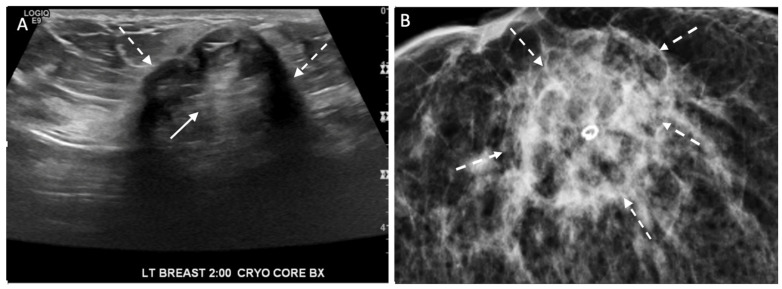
Ultrasound (A) and corresponding mammogram (B) of cryoablation site performed 6 months after cryoablation with broken arrows indicating outer edges of the cryoball and solid arrow (A) at site of central tumor ghost.
